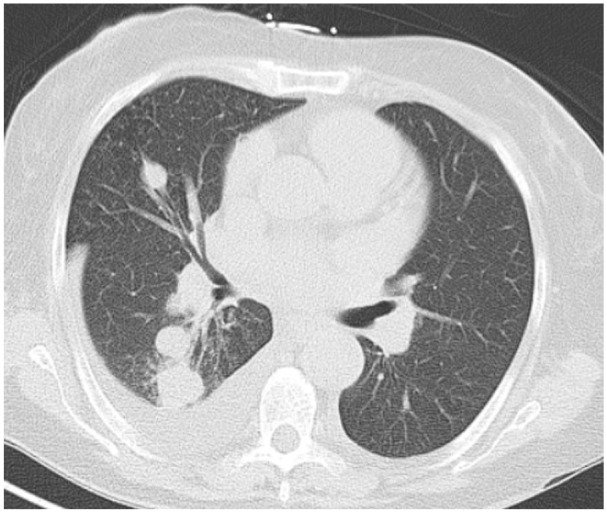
CT chest without contrast showed nodular lesions and a right-sided pleural effusion.
